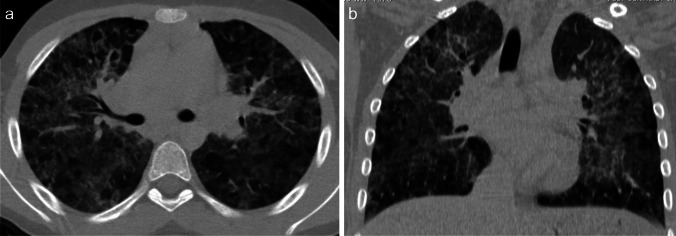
Axial (a) and coronal (b) contrast-enhanced lung window computed tomography images in a 9-year-old girl with a 3-month history of chronic dry cough, wheezing, and loss of weight demonstrate patchy bilateral ground-glass opacities, small centrilobular nodules, lobular areas of reduced attenuation and vascularization (i.e., mosaic attenuation), and interlobular septal thickening with sparing of the lung bases. No associated features of chronic lung disease. Lung biopsy confirmed hypersensitivity pneumonitis. On further investigation, her mother also had similar clinical symptoms. The family had started breeding pigeons 6 months prior. When the antigen was removed, the child’s symptoms completely resolved, and follow-up chest radiography performed 3 months later (not shown) was normal
